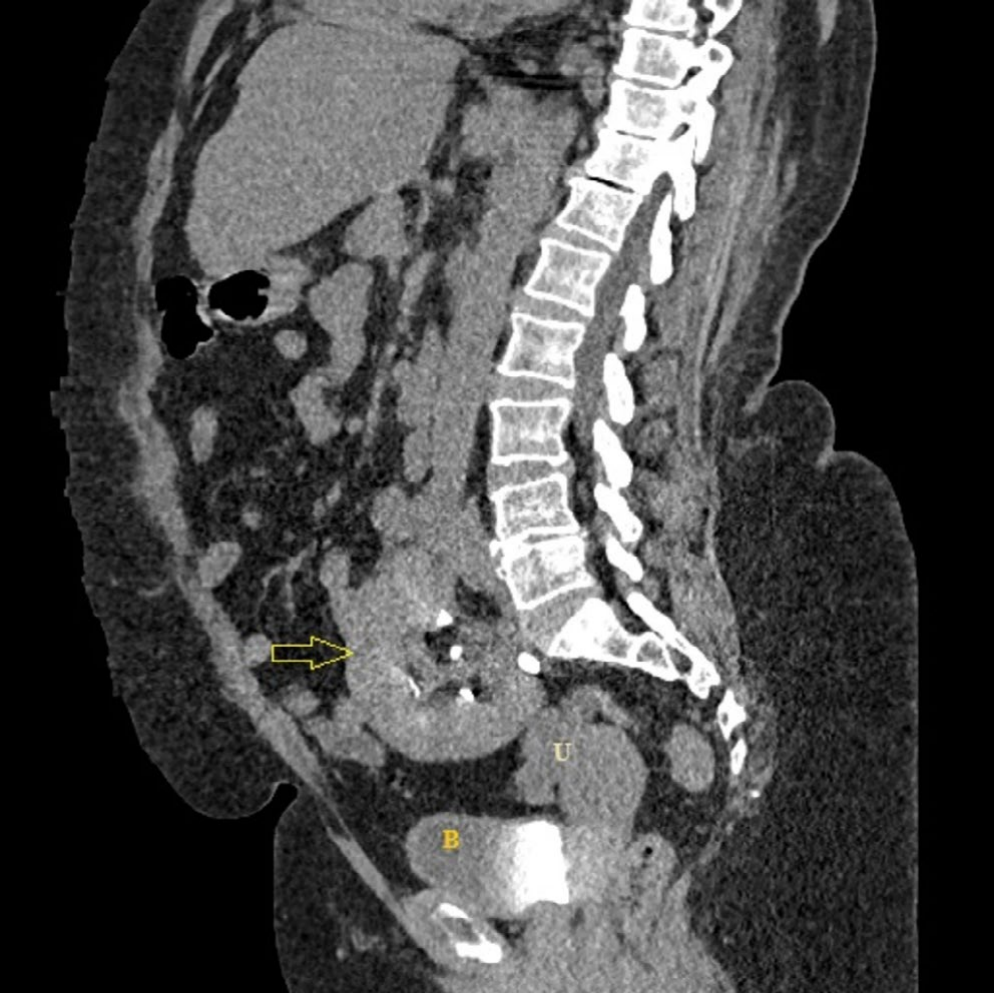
Sagittal view, contrast‐enhanced CT scan. This reconstruction demonstrates the ectopic kidney (yellow arrow) in the pelvis, at the level of L5–S1, having close contact with the uterus (U).
